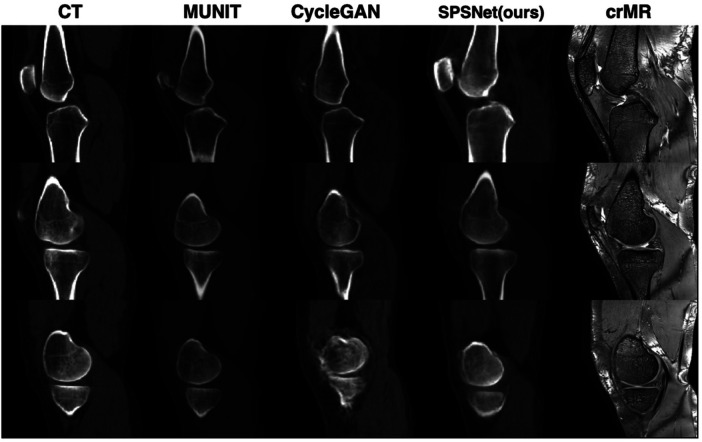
Comparison of crMR-to-sCT synthesis using different methods. Each row displays the synthesis results of a sampled slice from the test dataset. The columns, arranged from left to right, represent CT images, sCT images by MUNIT (30), sCT images by CycleGAN (31), sCT images by our proposed SPSNet and the original crMR images, respectively. crMR = coarsely-registered MR, sCT = synthesized CT.
